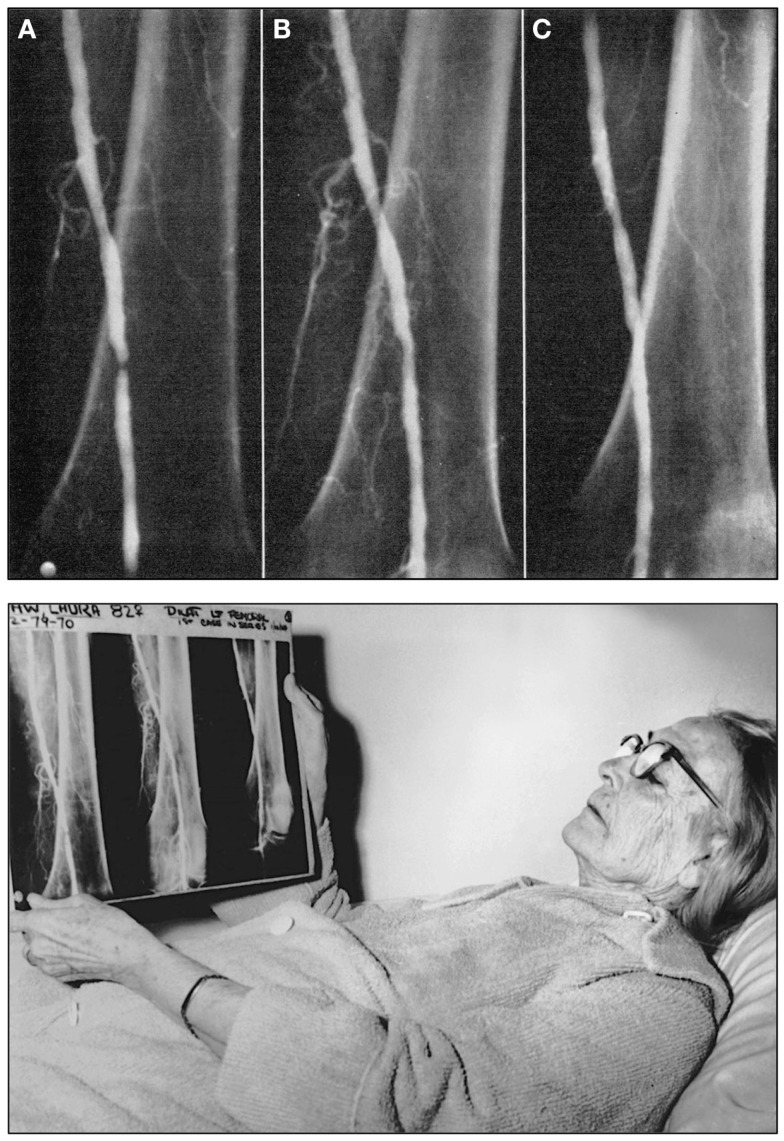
Top: Angiograms of the first intentional percutaneous transluminal angioplasty (PTA) performed on January 16, 1964 by Charles T. Dotter, M.D. and Melvin P. Judkins, M.D., in patient Laura Shaw. (A) The initial control angiogram reveals a tight, thread-like stenosis of the left distal superficial femoral artery. (B) Angiogram of the same artery immediately after the angioplasty procedure, which was performed using a 3.2 mm co-axial catheter (C) Follow-up angiogram of the same artery 3 weeks after the PTA procedure demonstrates luminal patency, indicating successful angioplasty. Bottom: Charles Dotter’s first angioplasty patient, 83-year-old Laura Shaw in February 1964 three weeks after the first PTA. The patient holds the radiographic films with the same three angiograms shown in the top panel of this figure. Top figure reproduced from Dotter and Judkins (60), with permission of the American Heart Association. Bottom figure reproduced with permission of the Dotter Interventional Institute, Oregon Health and Science University, Portland, OR, USA.
